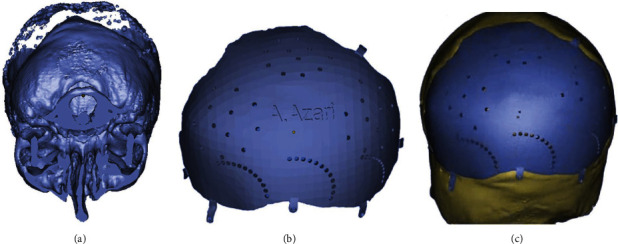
3D analysis. (a) CT scan shows the exact size and shape of the congenital defect. (b) The 3D virtual model of the prosthesis. (c) The 3D virtual model of the prosthesis adapted into the defect.
